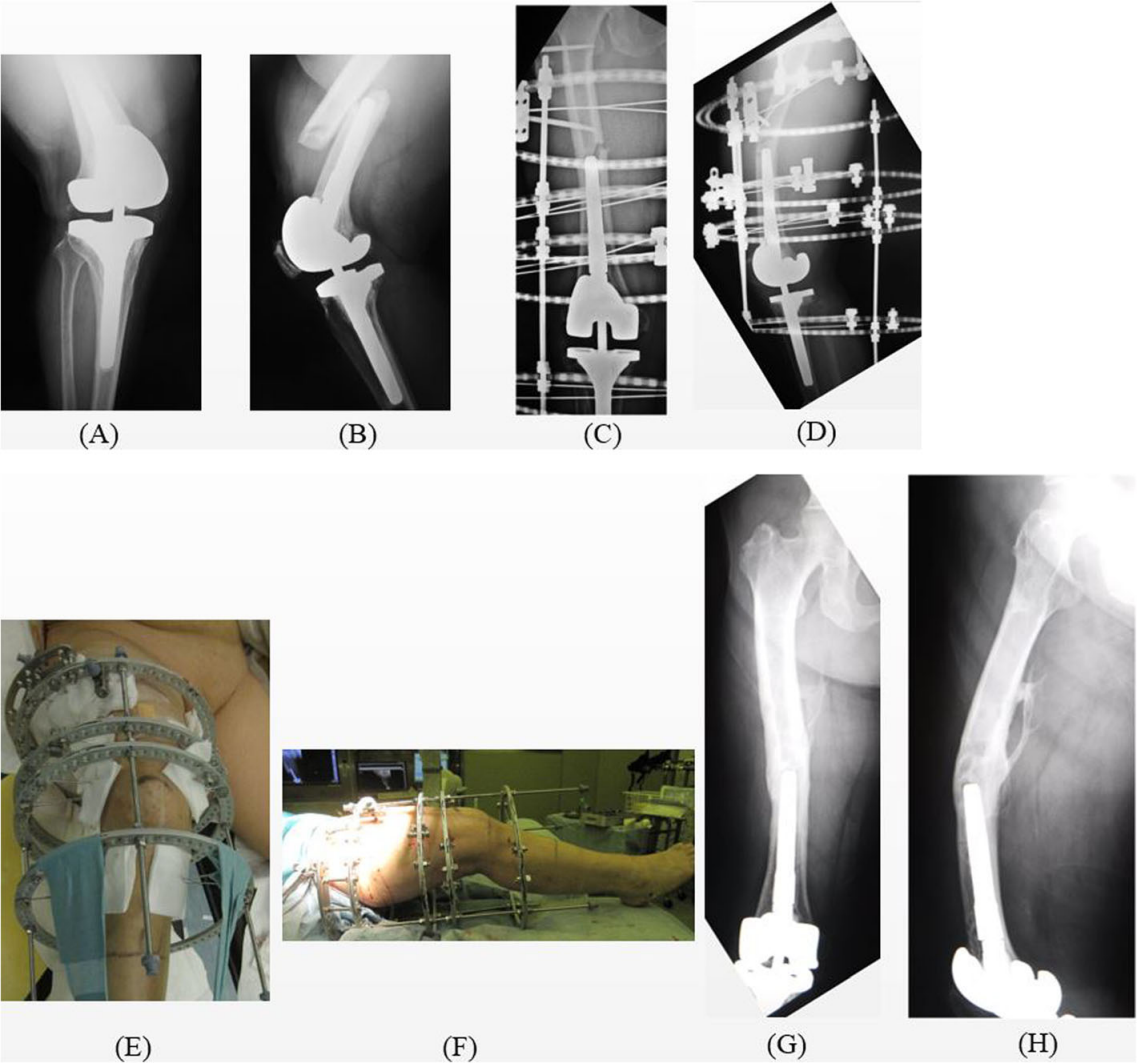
Periprosthetic distal femur fracture in case number 18 treated with a circular external fixator. (A) Preoperative antero-posterior X-ray. (B) Preoperative lateral angle X-ray. (C, D) Immediate postoperative X-ray. (E, F) Immediate postoperative clinical photograph. (G, H) Postoperative 5 years
